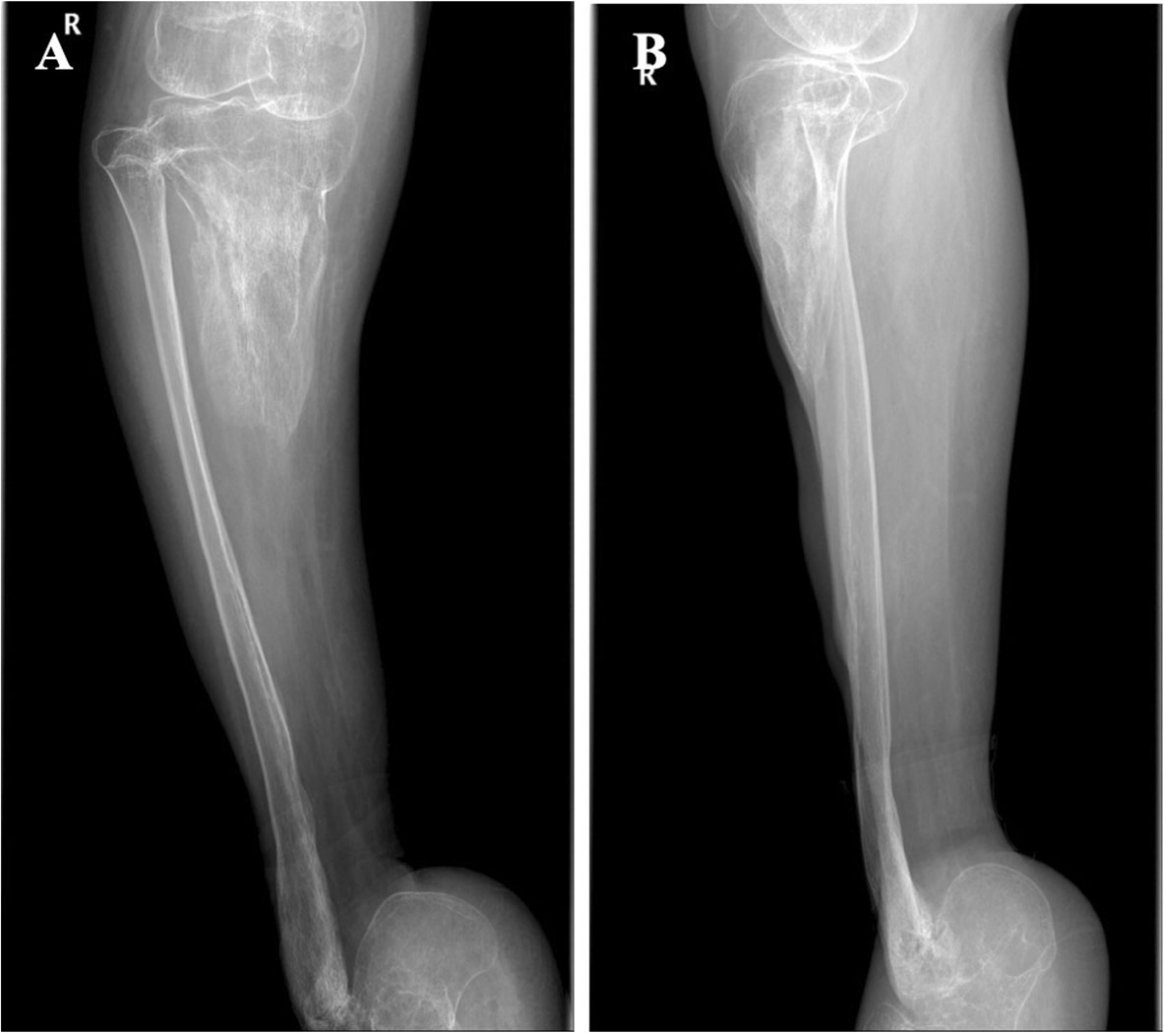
a, X-ray AP view of right lower leg. b, X-ray Lateral view of right lower leg
